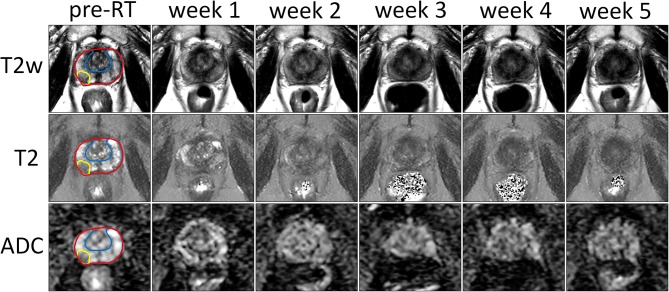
Example of T2-weighted images, and T2 and ADC maps of the prostate prior to treatment (pre-RT) and at each repeat MRI exam (weeks 1–5) of a patient treated at the NKI. The entire prostate, the boundary between PZ and CG and the tumor are delineated in red, blue, and yellow, respectively.
